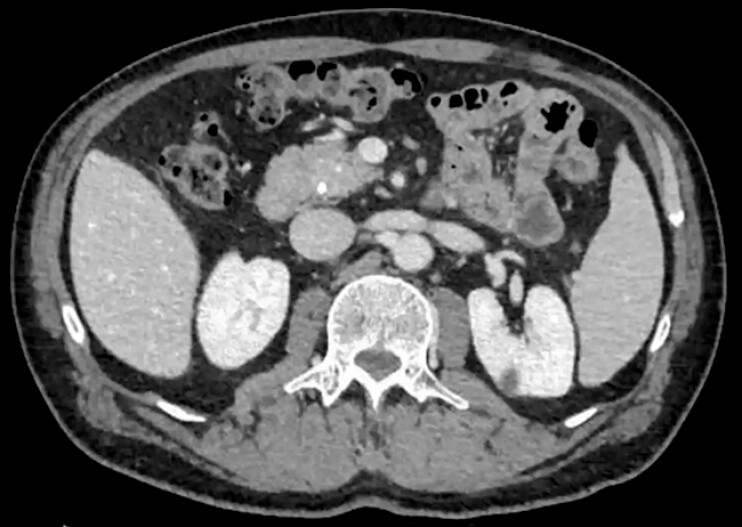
Abdominal computed tomography showing two retained stent fragments in the PD and mild PD dilatation.
